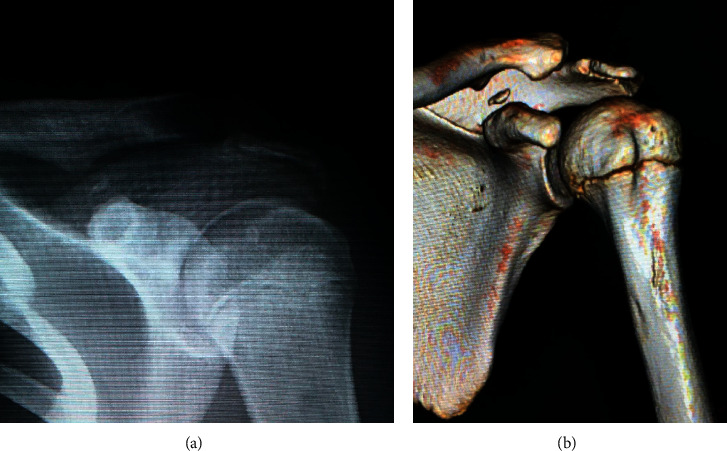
Representative anteroposterior radiograph of case 9: three-dimensional computed tomography reconstruction (a) and type II injury with an associated acromioclavicular dislocation (b).
